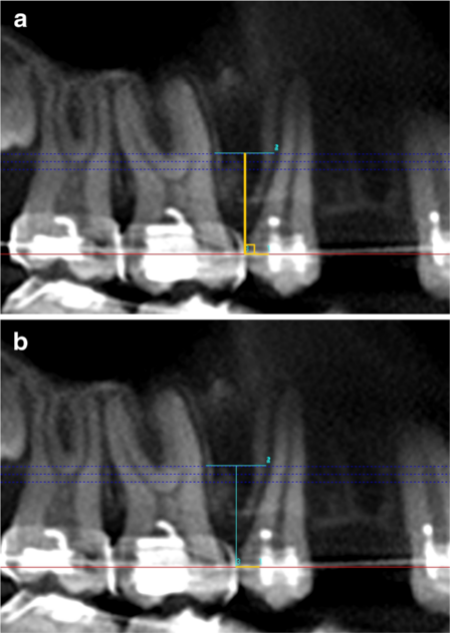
Determination of the point of entry of mini-implant by using CBCT data. (a) A perpendicular is dropped from ideal point of insertion of mini-implant to the arch wire. (b) Distance of point of intersection of perpendicular on the arch wire to distal end of second premolar bracket.The distance of the point of intersection of the perpendicular on the arch wire to the distal end of the second premolar bracket was measured (in the CBCT image) (Figure 3b).This distance was used clinically to mark the mesiodistal positioning of the mini-implant on the CBCT-guided side.The ideal height for the mini-implant placement was marked intraorally as determined in the CBCT image.
